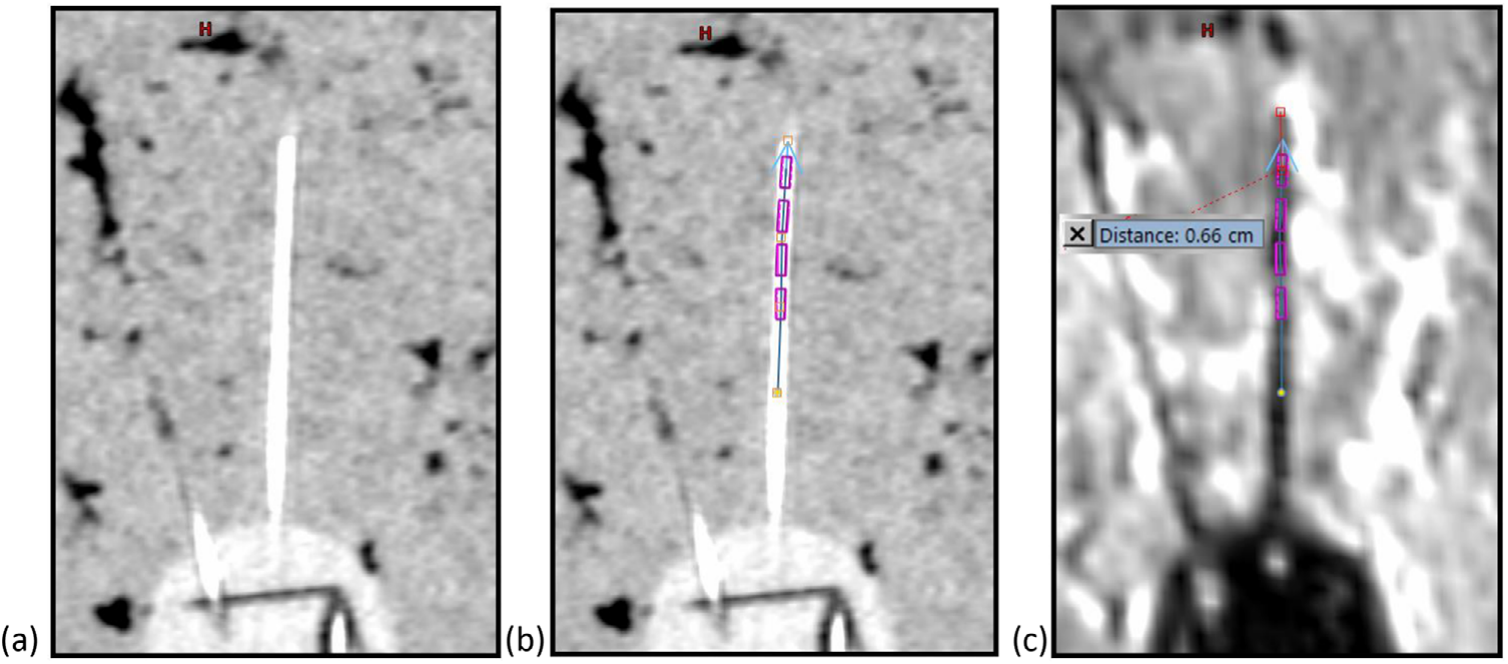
Signal void on MR images. After CT/MR image registration, an MR signal void is seen past the end of the digitized needle due to the physical dimension of the needle. Panel (a) shows an interstitial needle containing fish wire on CT image, (b) shows the digitized needle with the beginning of the needle placed at the end of the visible fish wire on CT images, and (c) shows the signal void beyond the tip of the digitized needle on T1 weighted MR image.
